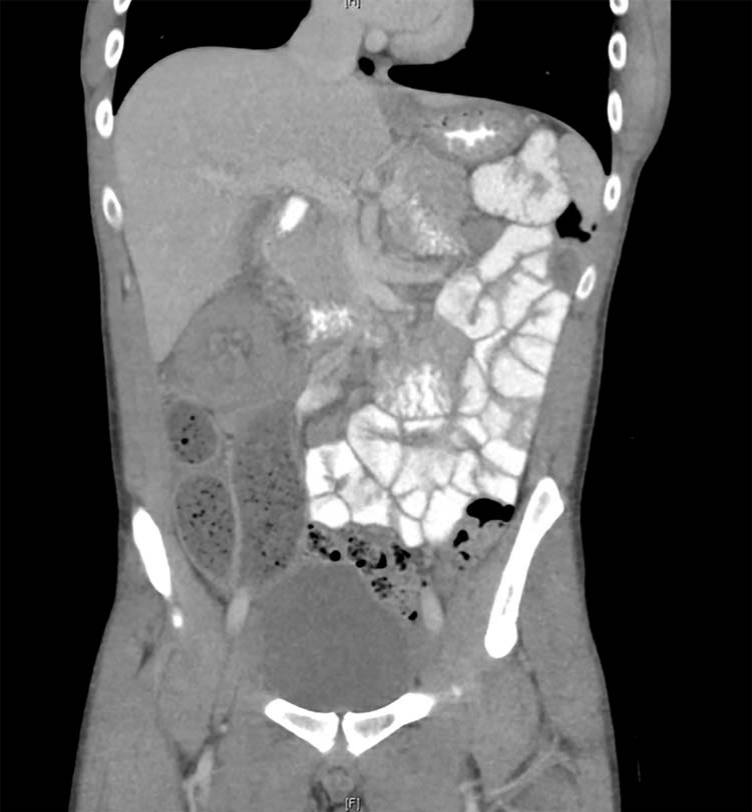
CT of the abdomen and pelvis with coronal view showing ileo-colic intussusception.
